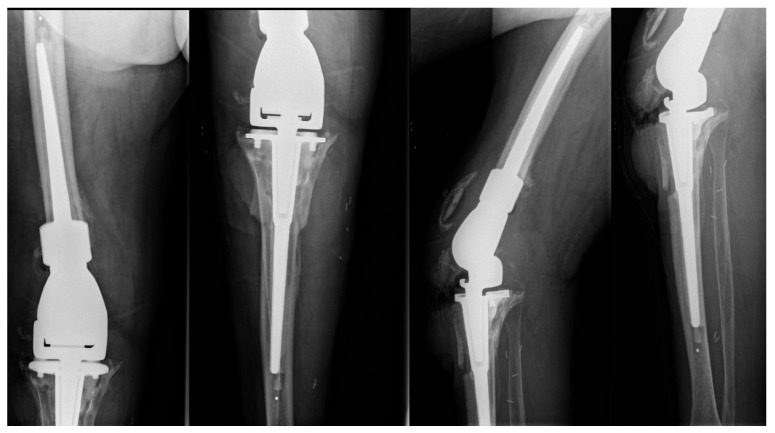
Anteroposterior and lateral X-ray views of the revised arthroplasty, which was performed prior to the patient’s presentation in the outpatient clinic.
